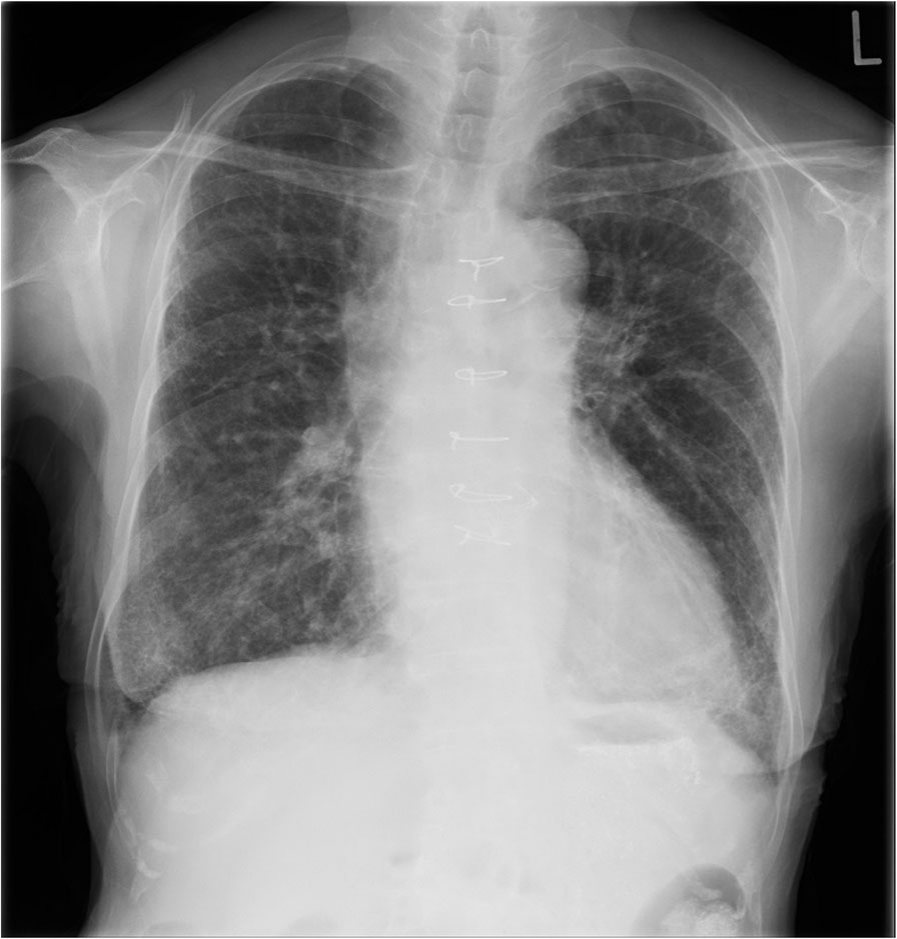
Chest radiography 12 months after AVR. Exacerbation findings were not confirmed in chest radiograph 12 months after AVR. *AVR* aortic valve replacement
